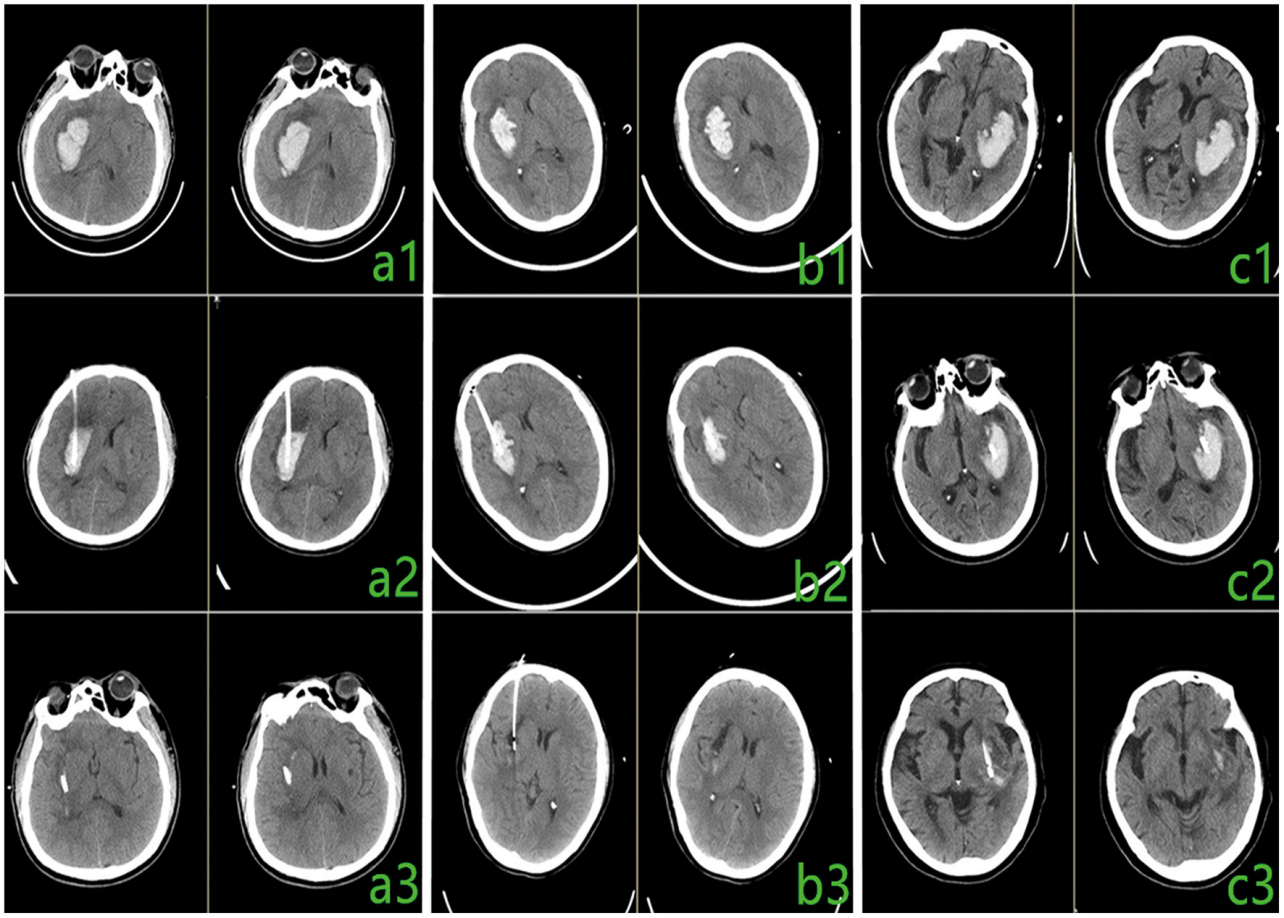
Comparison of preoperative and postoperative computed tomography (CT) results. (a1–c1) Preoperative CT. (a2–c2) Postoperative CT, 6 h after surgery. (a3–c3) Postoperative CT, 3 days after surgery.
